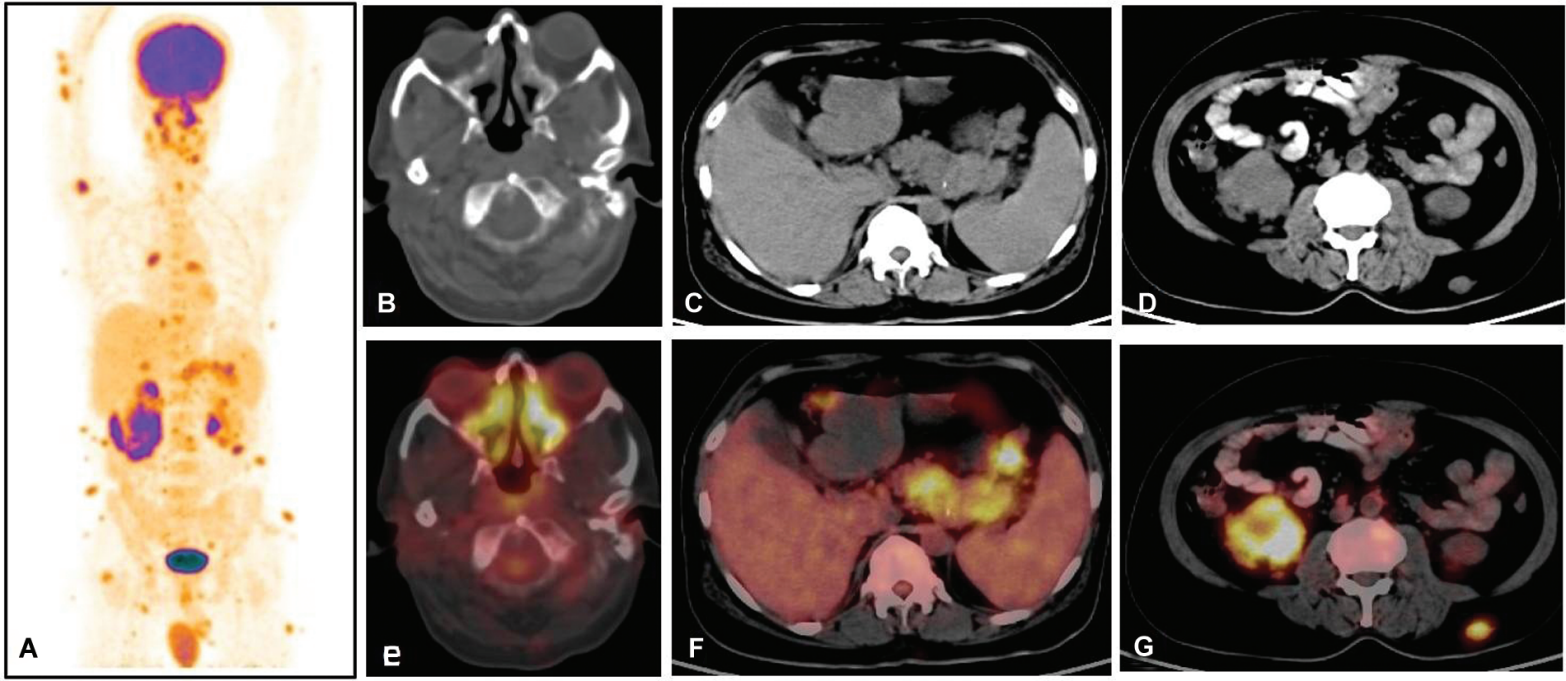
18F-fluorodeoxyglucose (F18-FDG) whole body positron emission tomography computed tomography (PET CT) maximum intensity projection image (
